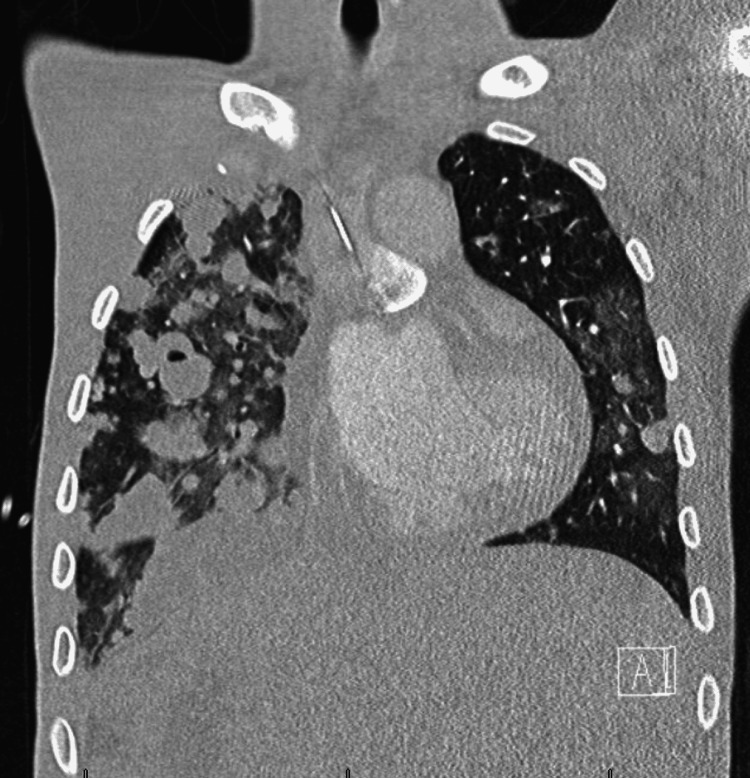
Repeat thoracic CT. CT chest showing innumerable lung lesions, which is worse than the previous studies raising concern for progressive metastatic disease
